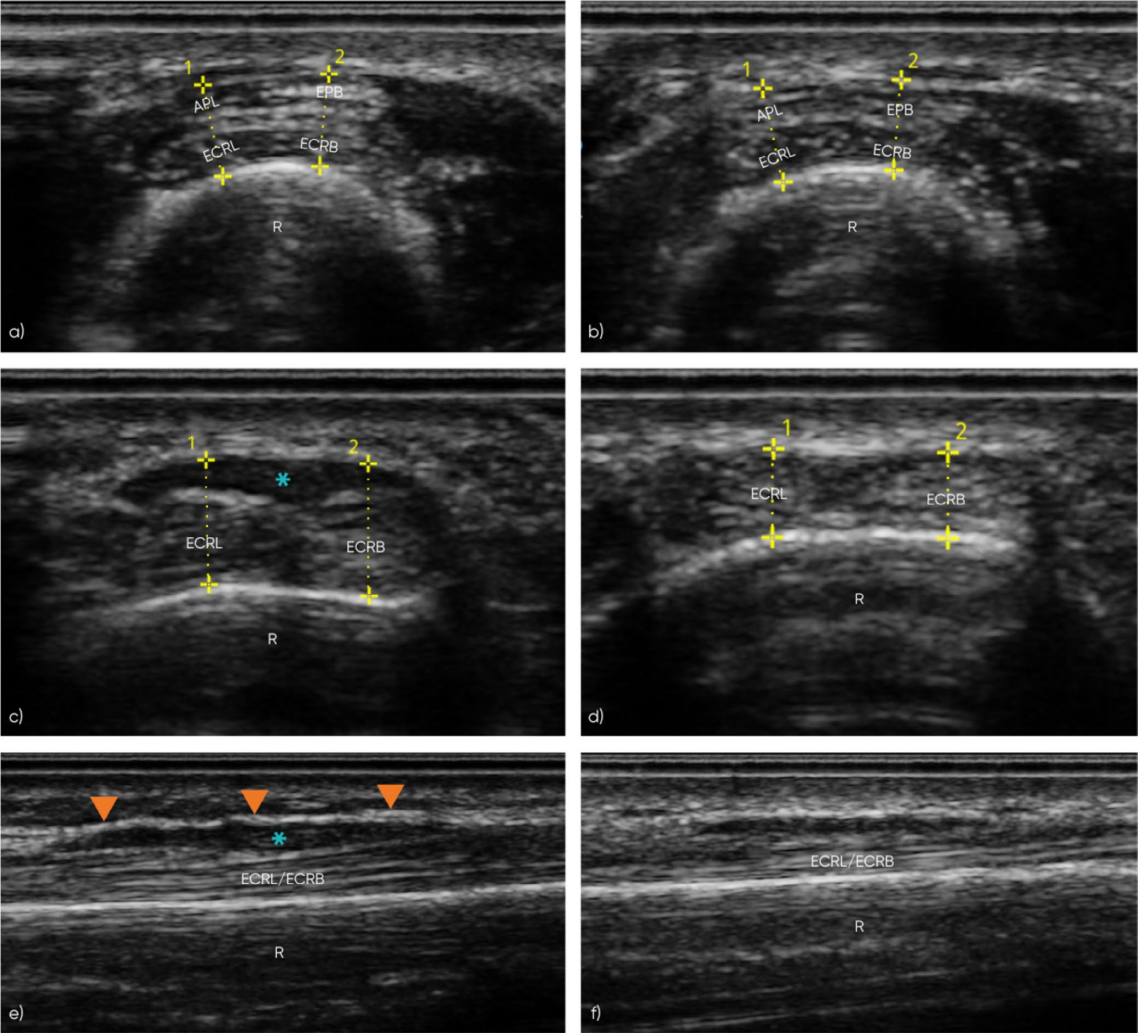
Ultrasound assessment of the symptomatic and asymptomatic limbs, at the proximal intersection, during the initial appointment. The figure displays short‐axis and long‐axis ultrasound images comparing the symptomatic (left column) and asymptomatic (right column) limbs. Short‐axis images at the proximal (a, b) and distal (c, d) sites of the proximal intersection highlight tendon morphology and sites of measurements, while long‐axis views (e, f) demonstrate EPB muscle characteristics. (a, b) Short‐axis images at the proximal site of the proximal intersection (ECRL‐APL and ECRB‐EPB). (a) Symptomatic limb shows normal tendon thickness and echogenicity. (b) Asymptomatic limb demonstrates similar normal findings. (c, d) Short‐axis images at the distal site of the proximal intersection (ECRL‐EPB and ECRB‐EPB). (c) Symptomatic limb shows mild tendon thickening and reduced echogenicity, consistent with tendinosis. (d) Asymptomatic limb displays normal tendon appearance and echogenicity. (e, f) Long‐axis views of the EPB muscle. (e) Symptomatic limb reveals a hypertrophied EPB muscle (blue asterisk) with thickened superficial connective tissue/fascia (orange arrowheads) and mild hypoechogenicity of the muscle, indicative of edema. (f) Asymptomatic limb shows normal EPB muscle morphology. *R*, radius; ECRL, extensor carpi radialis longus tendon; ECRB, extensor carpi radialis brevis tendon; APL, abductor pollicis longus tendon; EPB, extensor pollicis brevis tendon; blue asterisk, extensor pollicis brevis muscle; orange arrowheads, superficial connective tissue/fascia; yellow dotted lines with numbers, measurement sites for tendon thickness recorded during ultrasound scanning.
